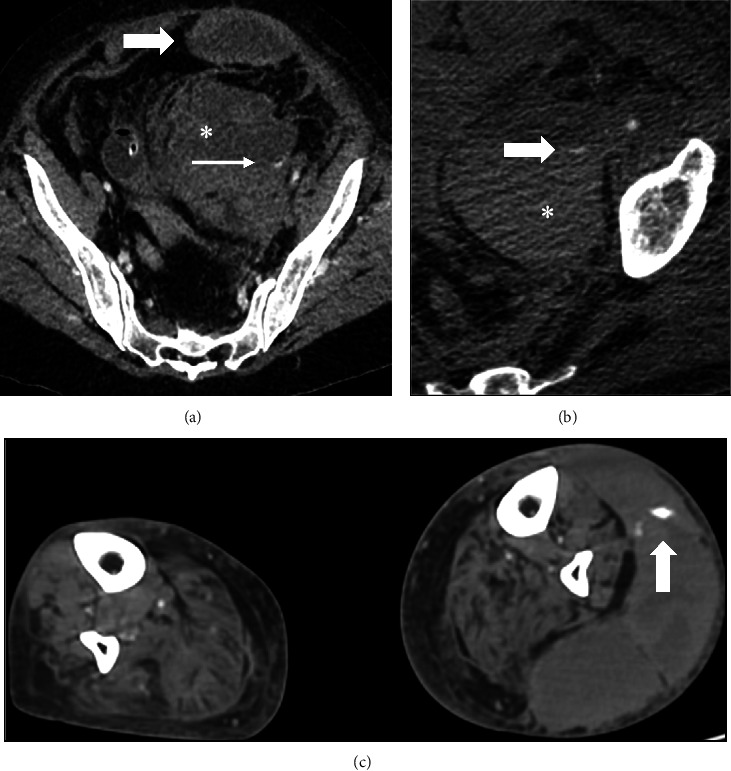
Angio-CT images of three exampled patients. (a) Left rectus sheath haematoma (gross arrow) with preperitoneal space extension (^*∗*^) and extravasation of contrast material (thin arrow). (b) Left retroperitoneal haematoma (^*∗*^) with extravasation of contrast material (arrow). (c) Left leg hematoma with active bleeding (arrow).
